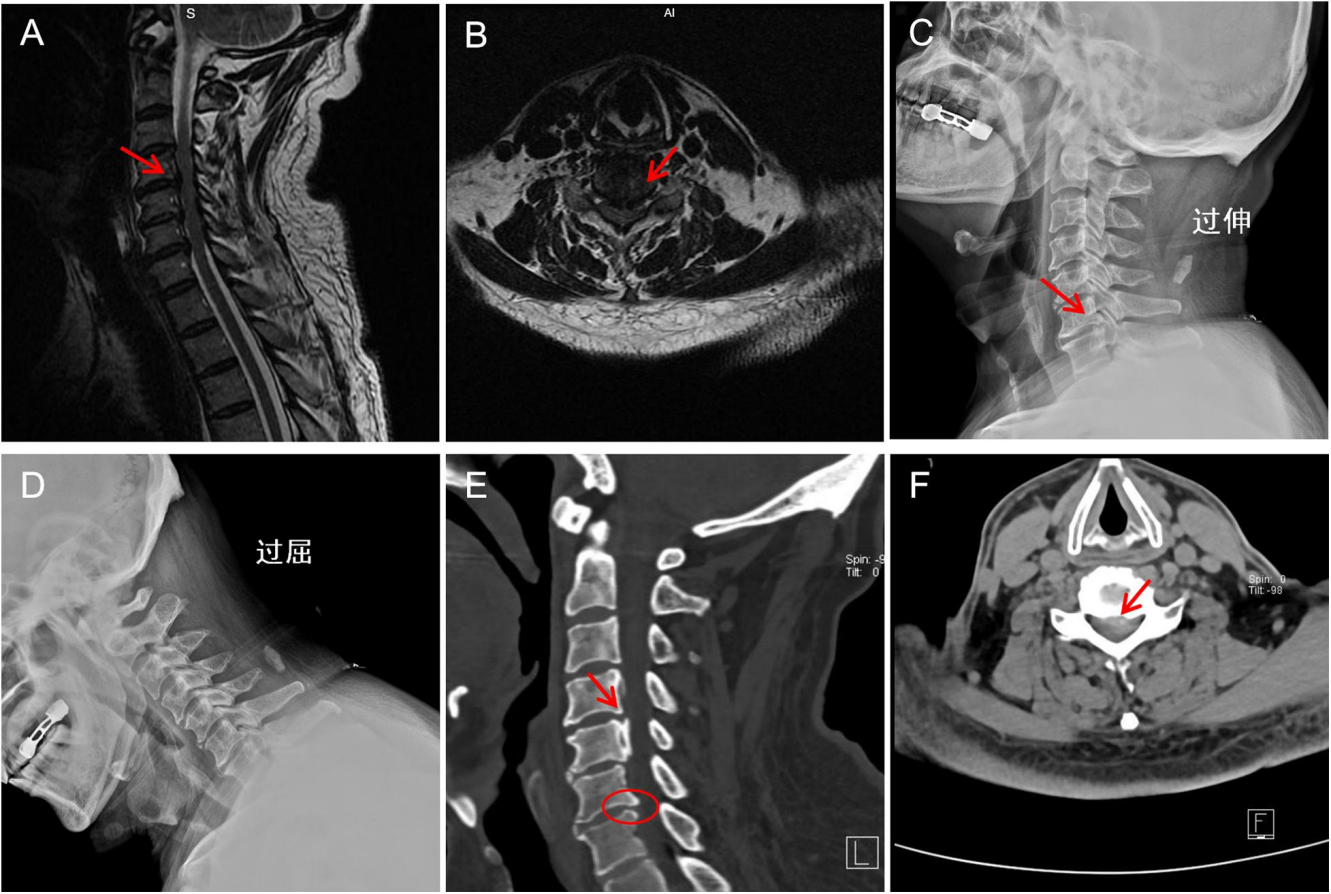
Preoperative imaging examinations of the patient. (A) Sagittal MRI showing cervical spinal canal stenosis with hypertrophy and proliferation of the posterior longitudinal ligament and ligamentum flavum. (B) Axial MRI image showing significant spinal cord compression. (C) Extension-position dynamic radiograph showing straightening of the normal cervical lordosis and osteophyte formation at the posterior margin of the C6/7 vertebral body. (D) Flexion-position dynamic radiograph revealing cervical instability. (E) CT image showing ossification of the posterior longitudinal ligament at the C4 and C5 vertebral levels. (F) CT image showing disc herniation at C4–C6 with spinal cord compression.
